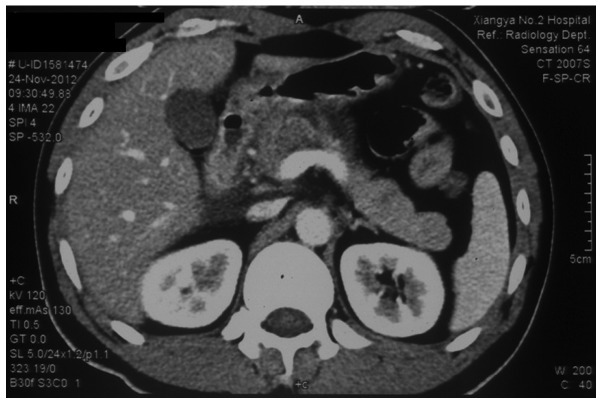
Computed tomography scan demonstrating diffuse swelling of the pancreas and peripancreatic fluid exudation.
